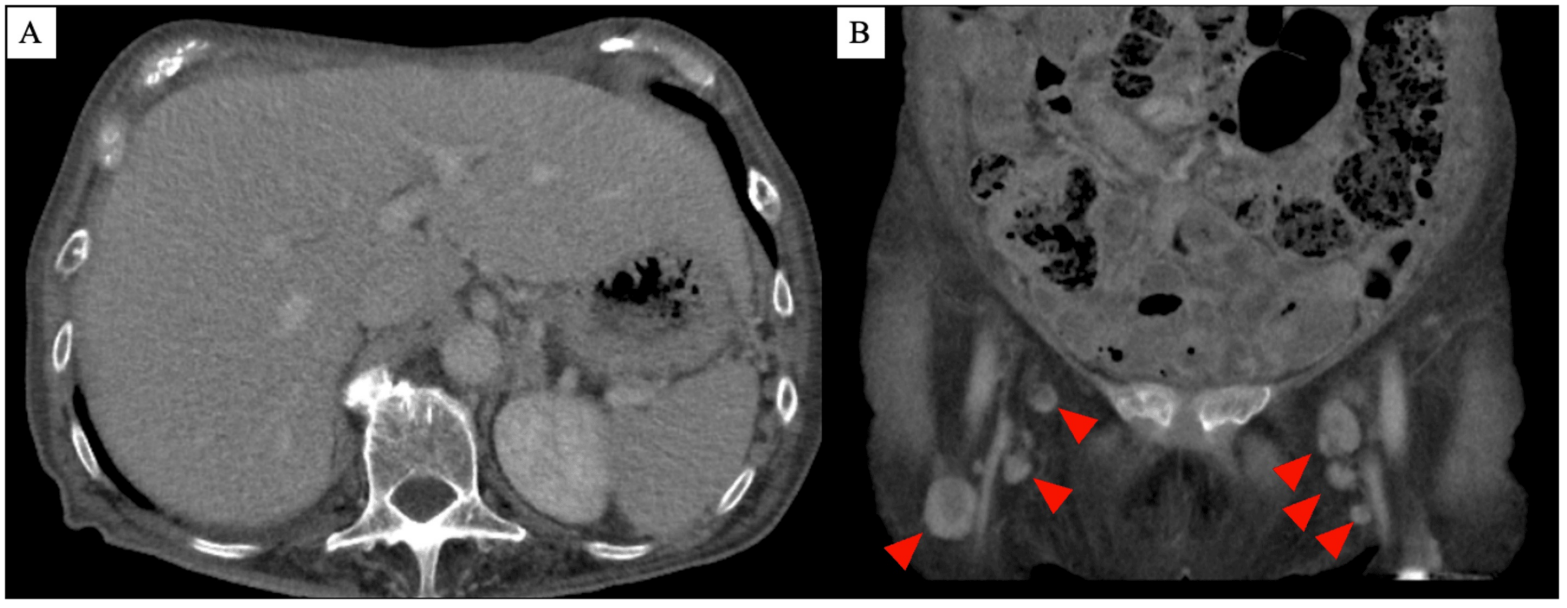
CECT of the abdomen and pelvis (A) Abdominal CECT revealed liver enlargement; (B) Pelvic CECT of the pelvis revealed lymph node enlargement in both inguinal regions (red arrowheads). CECT, contrast-enhanced computed tomography
